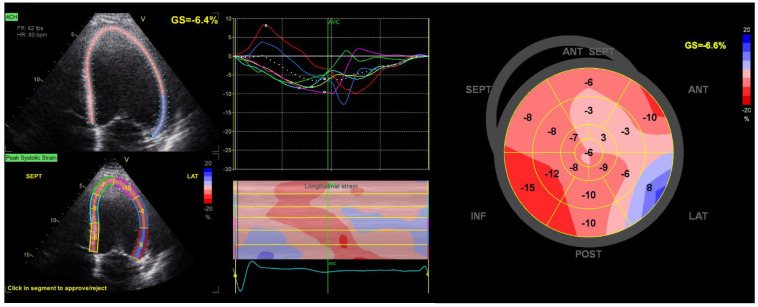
Global and regional strain. The figure shows an example of strain analysis in a patient with ischemic cardiomyopathy and ICD who experienced appropriate therapy after implantation. On the left side, speckle tracking of the left ventricle in the apical 4–chamber view is shown. In the middle panel, strain profiles for each segment are shown (colored curves), and the global value for this projection is shown as the white dotted profile. On the right, a corresponding bulls–eye plot of regional strain values is shown, and the global strain value is denoted as ‘GS’ in the upper right-hand corner. Overall, this patient had markedly reduced global longitudinal strain and widespread regional abnormalities in longitudinal strain, most notable for the basal lateral wall segment, showing positive strain values indicating paradoxical motion.
